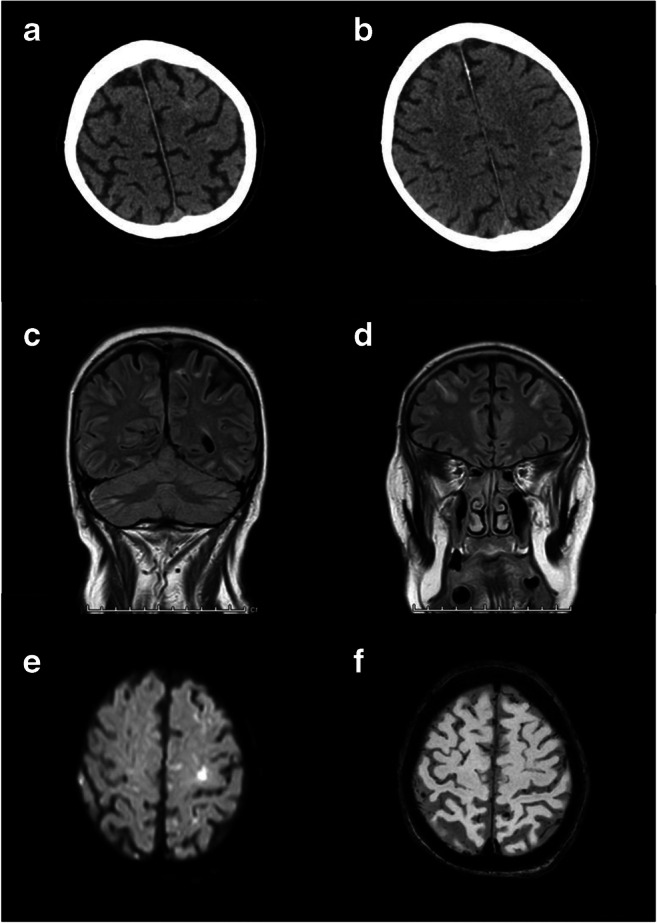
Non-aneurysmal SAH co-existent with COVID-19 (MRI). In patient A (a–f), brain MRI results demonstrated multifocal convexity high signal change consistent with acute subarachnoid haemorrhage and small infarcts including in the left precentral gyrus (a and b axial T1, c and d coronal fluid-attenuated inversion recovery, e axial diffusion, f axial susceptibility weighted imaging)
